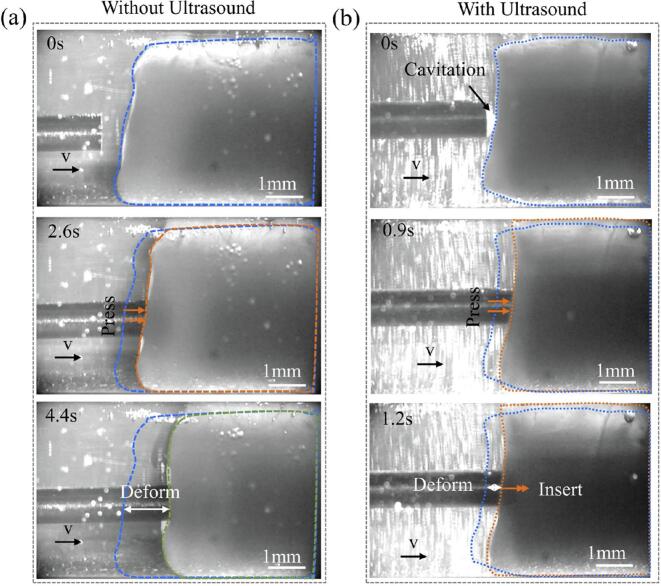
Comparison of guidewire interaction with thrombus. (a) Without ultrasound and (b) with ultrasound.
